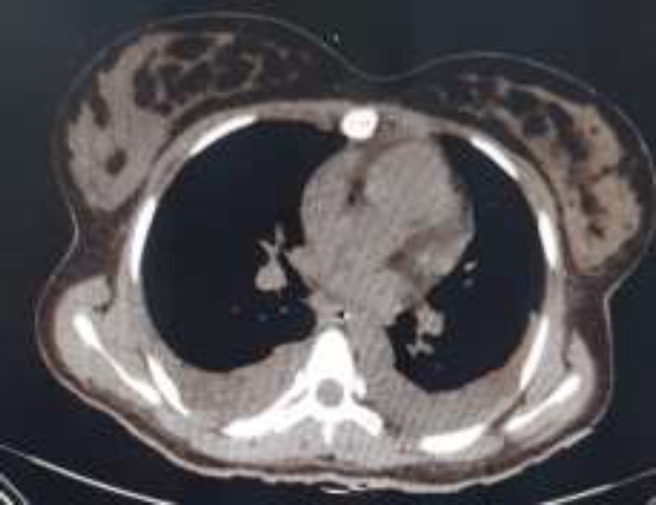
Chest cuts of abdominal CT scan revealed no findings suggestive for covid-19 pneumonia
